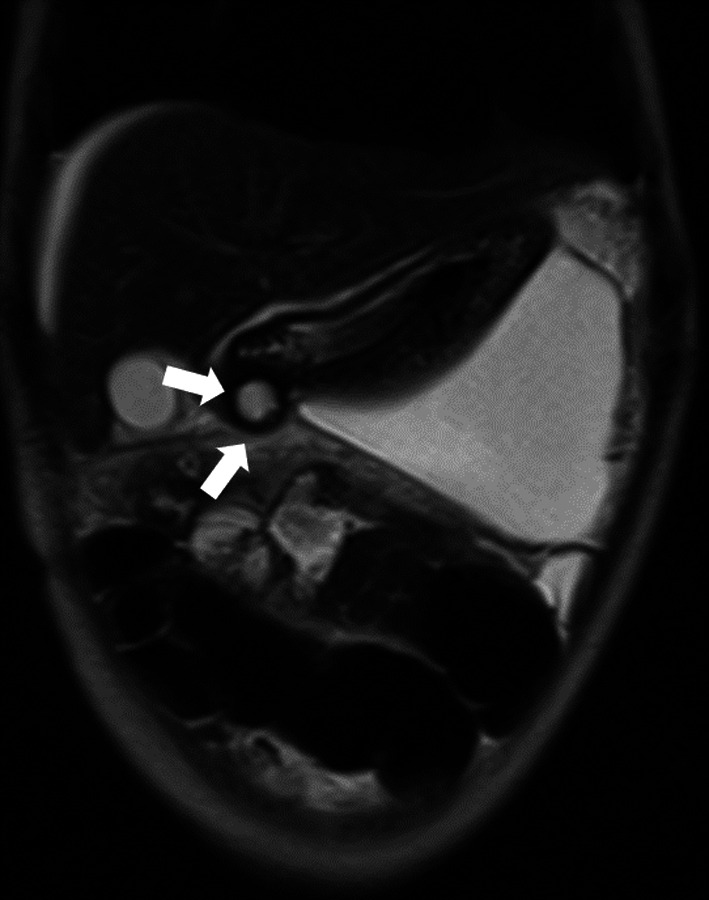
MRI findings. Magnetic resonance imaging showed that the cystic lesion (white arrow) became smaller with increased ascites mainly in the left upper abdomen. The pancreas itself had a normal appearance
